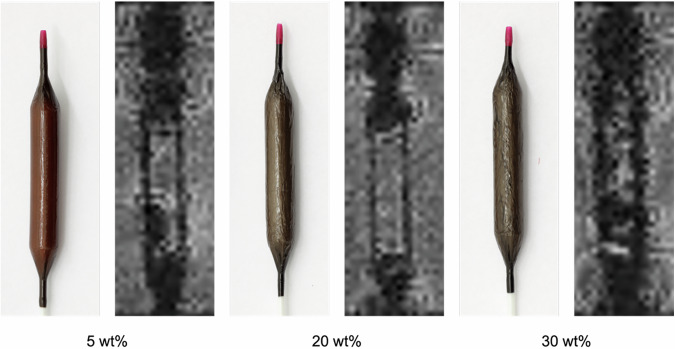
Photographs of balloon catheters with three SPION concentrations (5 wt%, 20 wt% and 30 wt%) with corresponding MR images. The greyish-brown colour of the balloons is caused by the iron oxide particles. A colour gradient with rising SPION concentrations towards darker brown tones is observed
